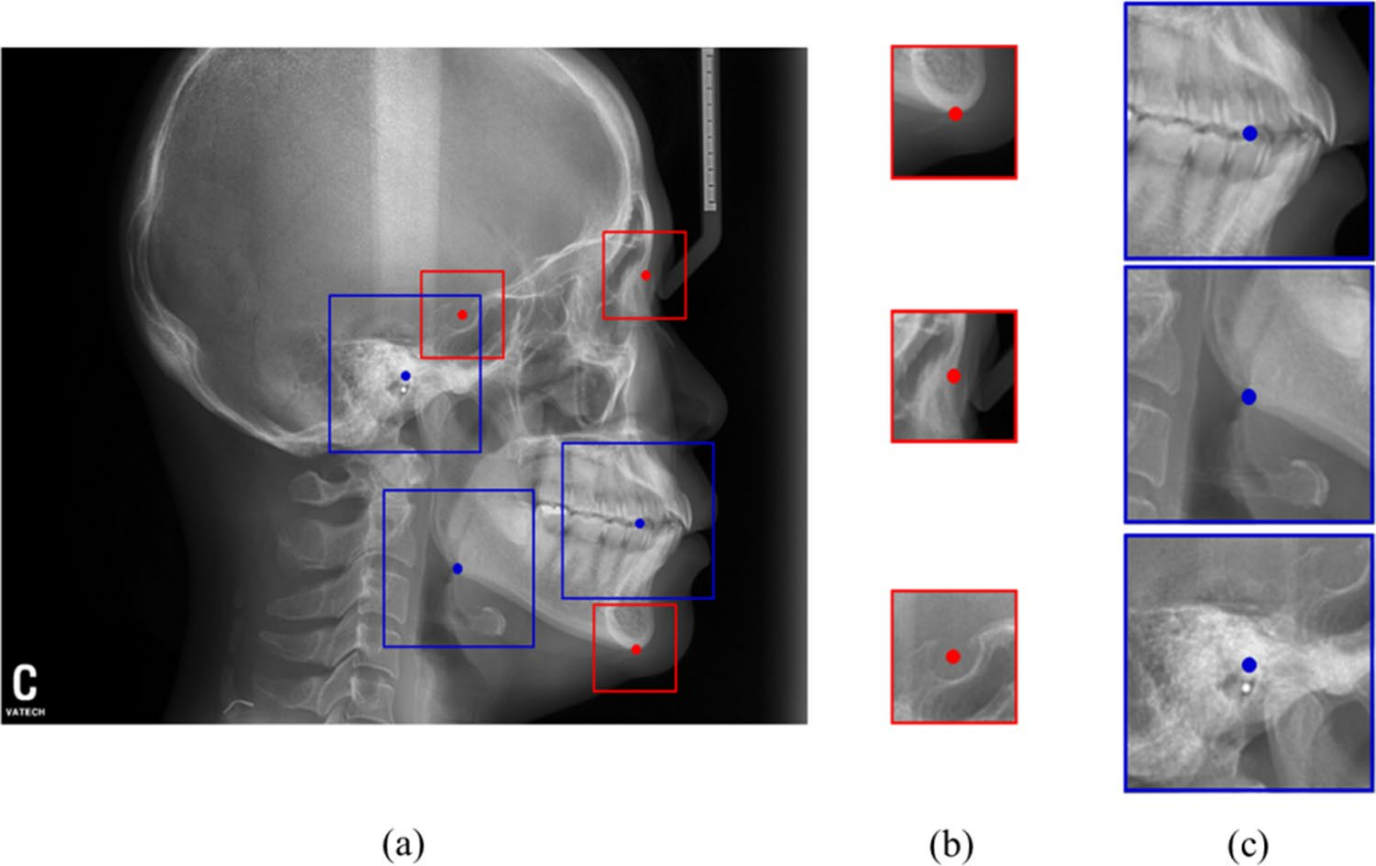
Two sizes of ROIs in the cephalometric X-ray. (a) ROIs with 256 × 256 and 512 × 512 size were extracted by landmarks. (b) Sella, nasion, and menton requiring a small ROI with 256 × 256 size (red), and (c) hinge, corpus and Md6 root requiring a wide ROI with 512 × 512 size (blue).
